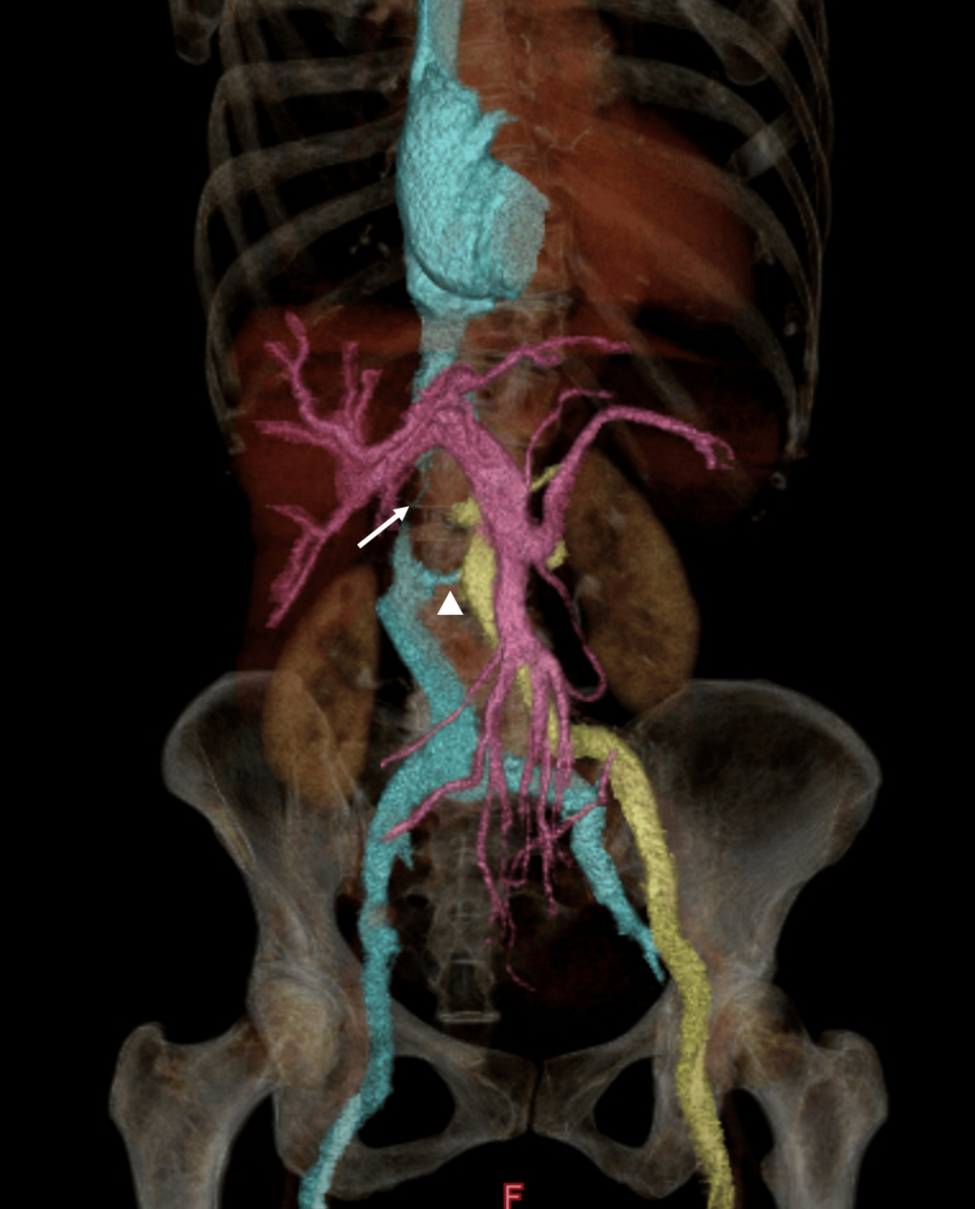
Three-dimensional CT reconstruction of venous system anomalies IVC: inferior vena cava 3D CT reconstruction of duplicated IVC and hepatic segment interruption. Yellow indicates the left-sided IVC, blue indicates the right-sided IVC, and red indicates the hepatic vein. The arrow highlights the interruption of the hepatic segment, the triangle indicates the left renal vein, through which the left-sided IVC drains into the right-sided system
